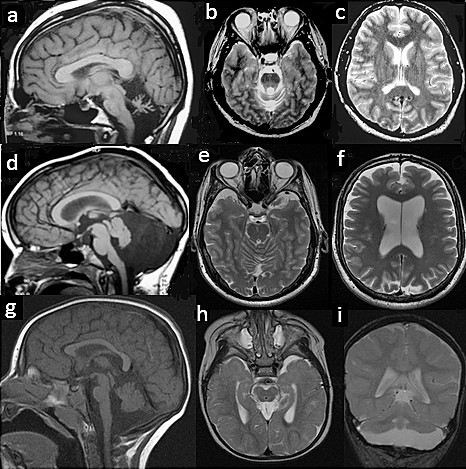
Brain MRI studies of patients with XLCD. 1 a-c (male proband of a family with X-linked congenital ataxia) Midsagittal T1-weighted image showing an overall size reduction of the cerebellum predominant in the vermis with widened interfolia sulci. Brainstem is normal (a). Axial T2-weighted image showing global cerebellar hypoplasia without dysplasia (b). Axial T2-weighted image showing normal cortex and ventricles (c). d-f (male patient carrying a *OPHN1 *mutation): midsagittal T1-weighted image showing hypoplastic cerebellar vermis and enlarged cisterna magna (d) axial T2-weighted image showing cerebellar hypoplasia and vermis dysplasia (e) axial T2-weighted image showing lateral ventricle dilatation and mild cortical atrophy (f). g-i (female patient carrying a *CASK mutation*): Midsagittal T1-weighted image showing moderate vermis hypoplasia and flat pons (g). Axial T2-weighted image showing simplified gyral pattern and ventricular dilatation (h). Coronal T2-weighted image showing global cerebellar hypoplasia and simplified cortical gyri (i).
